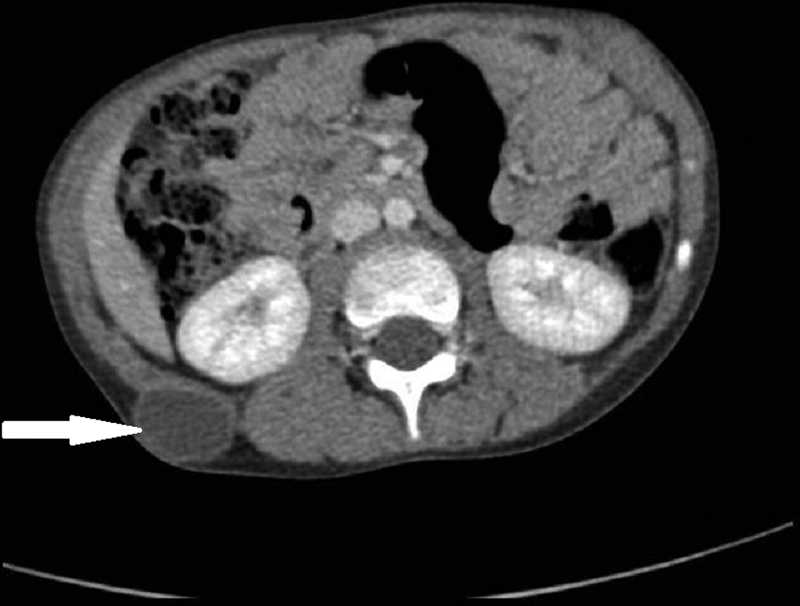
Abdominal computed tomographic scan showing a well-defined cystic mass of the subcutaneous soft tissues of the right posterolateral abdominal wall (arrow).
